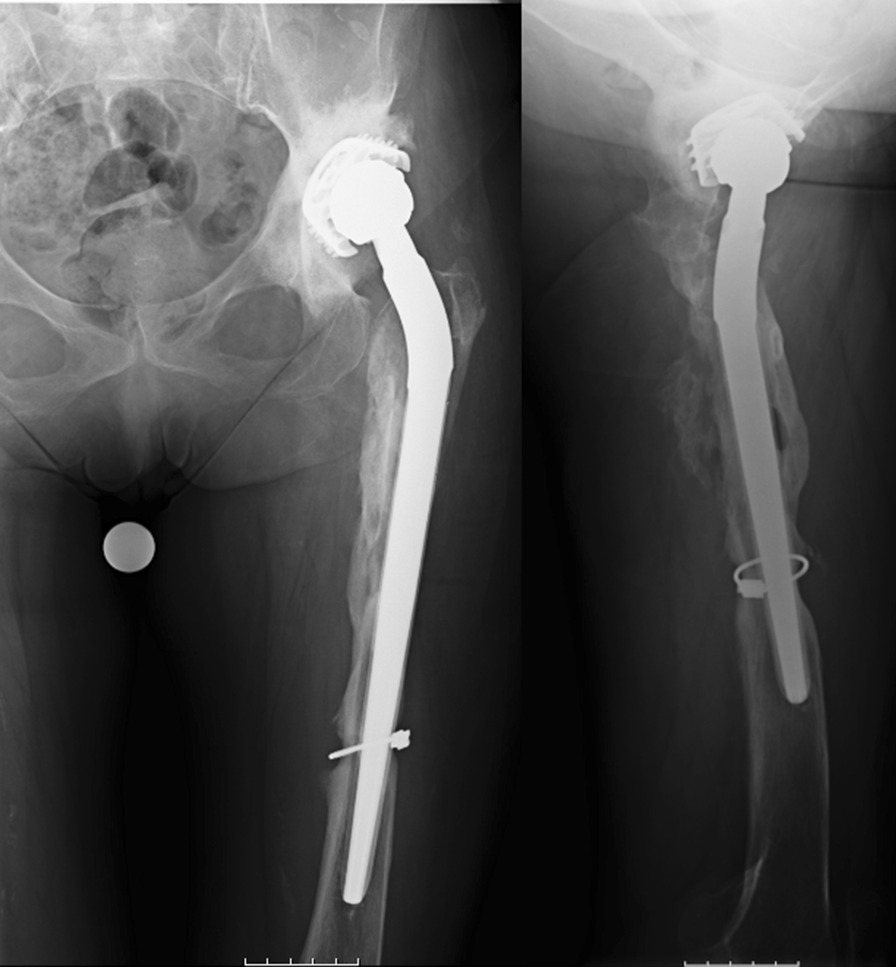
Case no. 20, preoperative X-rays showing the loosened implant surrounded by osteomyelitic bone
